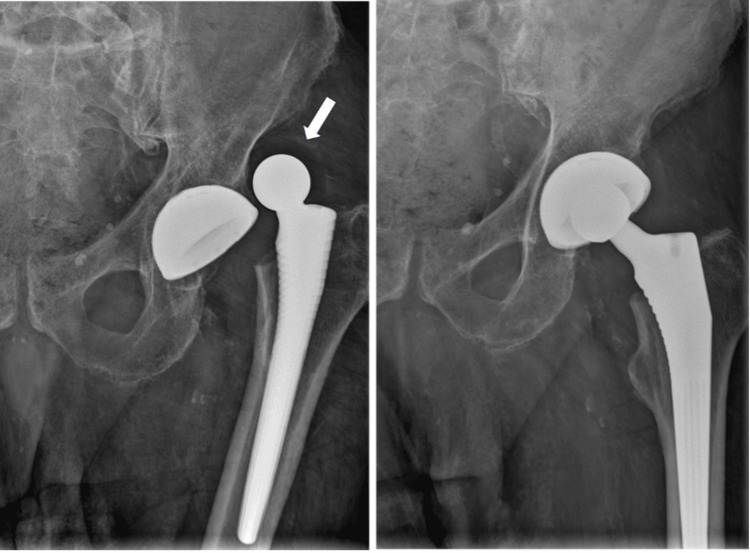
Hip radiographs obtained 16 days postoperatively demonstrating dislocation of the dual-mobility construct, with the femoral head-liner displaced from the acetabular cup (arrow).
